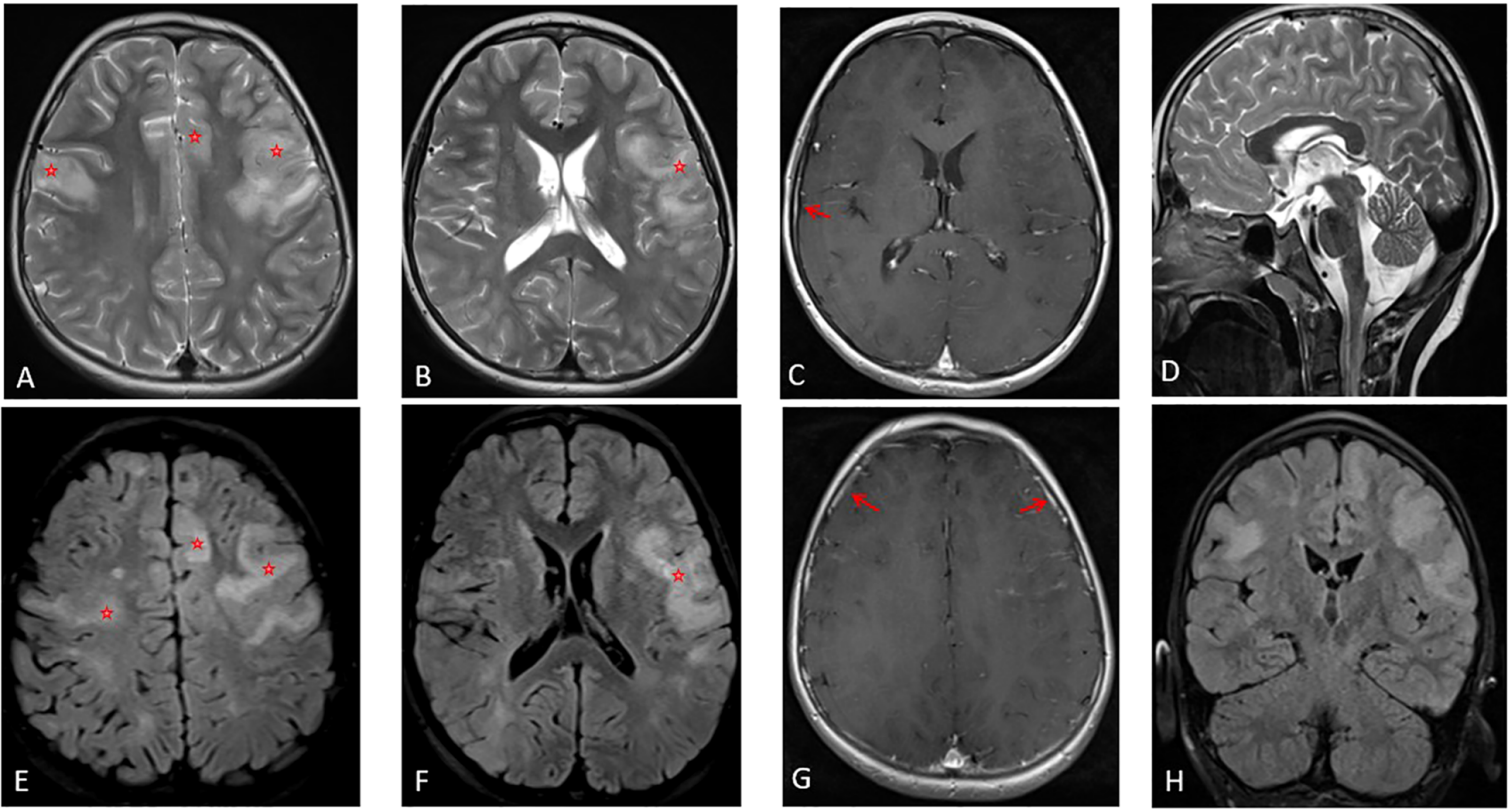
Cerebral MRI findings. Axial T2-weighted (A, B), axial FLAIR (E, F), and coronal FLAIR (H) images show bilateral cortical and subcortical hyperintensities with cortical swelling in the frontal and parietal lobes. Patchy contrast enhancement is visible on axial T1-weighted images with gadolinium (C, G). Sagittal T2-weighted (D) demonstrates the absence of the previously reported diencephalic and brainstem hypersignal.
